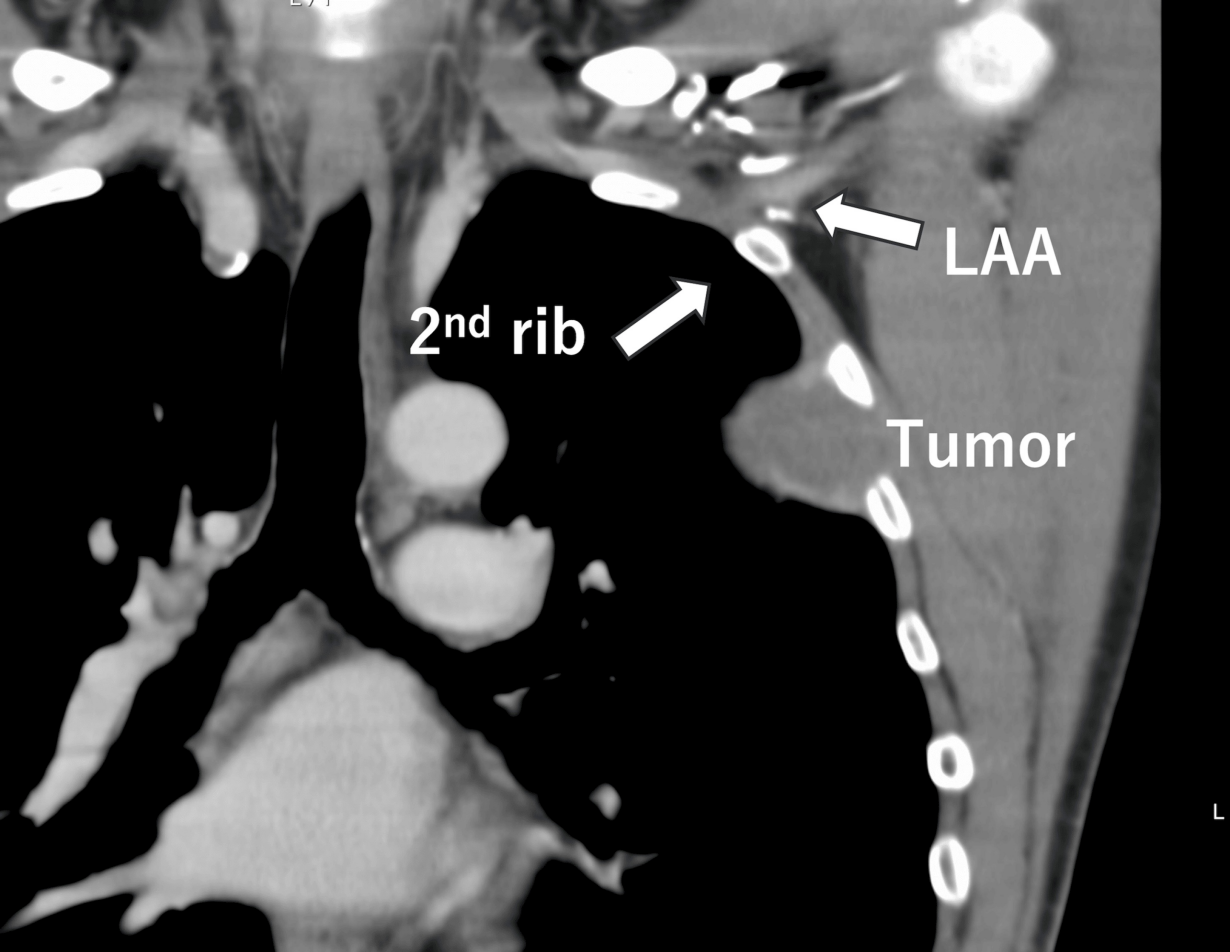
Retrospective review of the preoperative CT The LAA runs close to the superior border of the second rib. LAA: left axillary artery, CT: computed tomography
